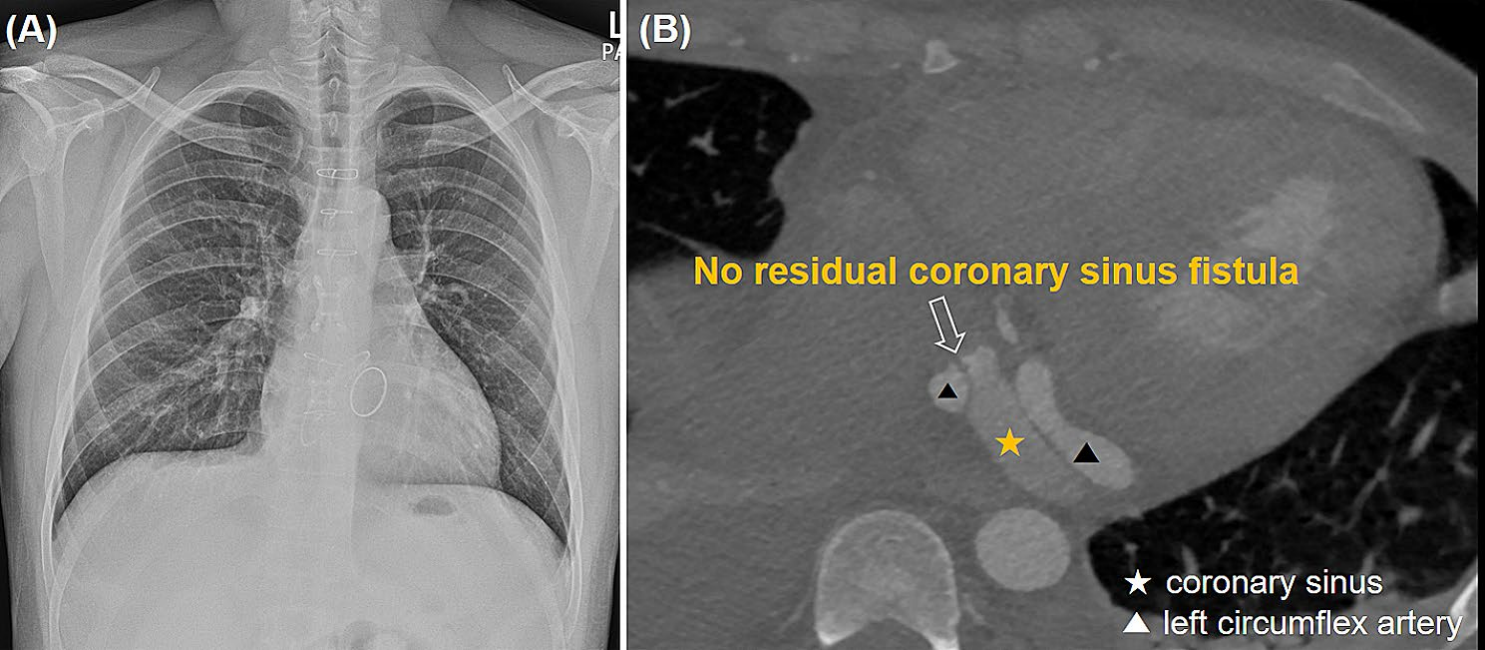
Postoperative images; (A) postoperative chest x-ray, (B) postoperative cardiac computed tomography indicated no residual (white blank arrow) fistula between LCx and CS
